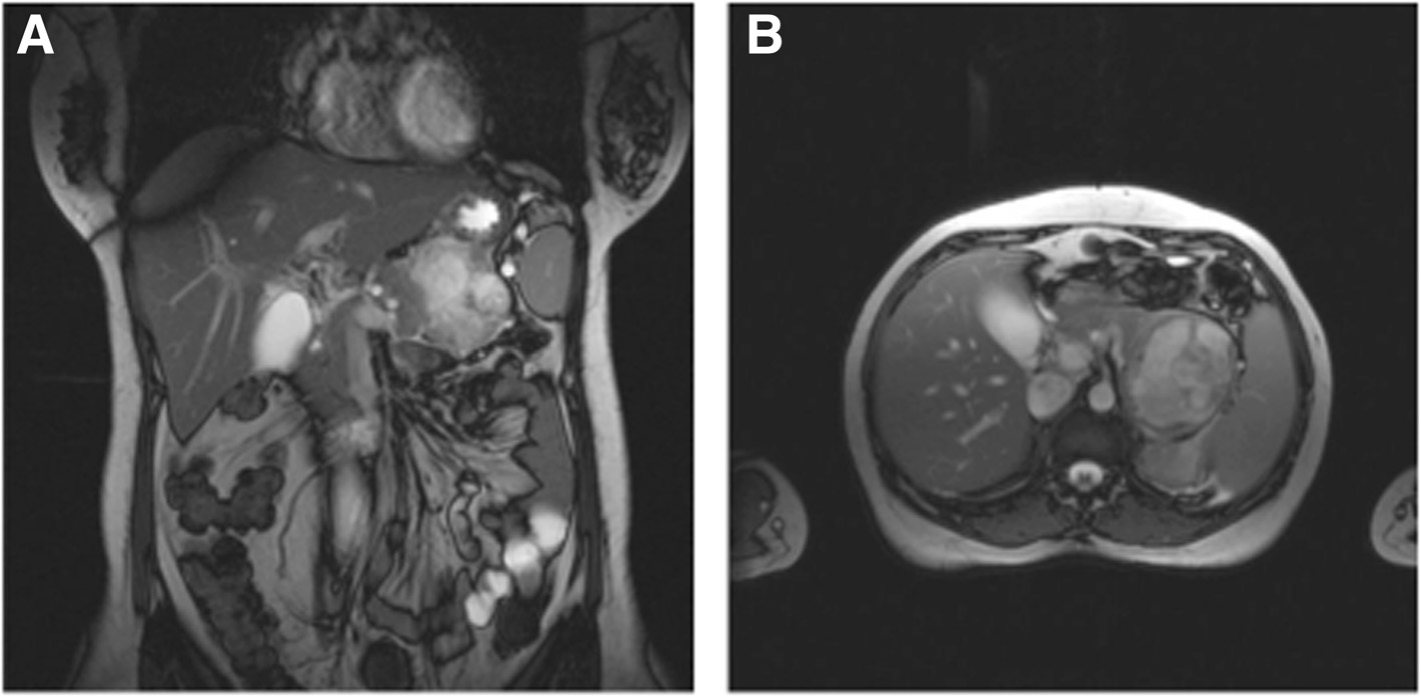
MRI of the upper abdomen showing the lesion in the pancreatic tail. (A) View in coronal plane. (B) View in axial plane.
